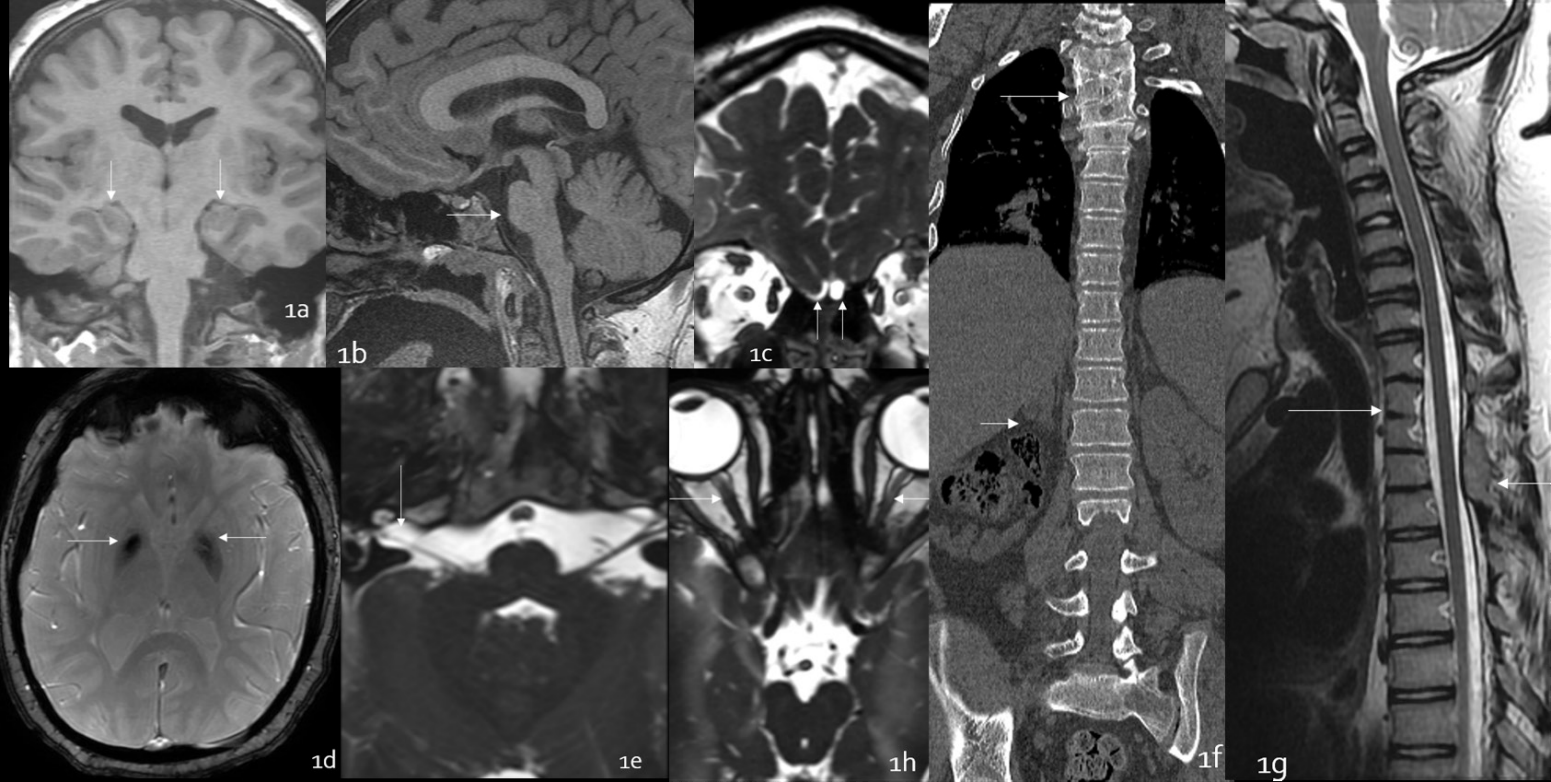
15-year-old male with TMCO1 mutation presenting with seizures. Coronal T1 (1a) demonstrates bilateral hippocampal malrotation (arrow); sagittal T1 FSPGR (1b) demonstrates pontine hypoplasia (arrow) ; coronal fast imaging employing steady state acquisition sequence (1c) demonstrates absence of bilateral olfactory bulbs and hypoplastic olfactory sulci (arrow); axial SWI sequence (1d) demonstrates abnormal susceptibility in globi pallidi (arrow) and axial fast imaging employing steady state acquisition sequence (1e) demonstrates absent right cochlear nerve (arrow); coronal CT bone window reformats (1f) and sagittal T2 (1g) demonstrates thoracic vertebral segmentation abnormality (long arrow), normal spinal cord and absent right kidney (short arrow). Fast imaging employing steady state acquisition sequence demonstrates hypoplastic bilateral optic nerves (1 h). FSPGR, fast spoiled gradient-echo; SWI, susceptibility-weighted imaging; TMCO1, transmembrane and coiled-coil domains one protein.
